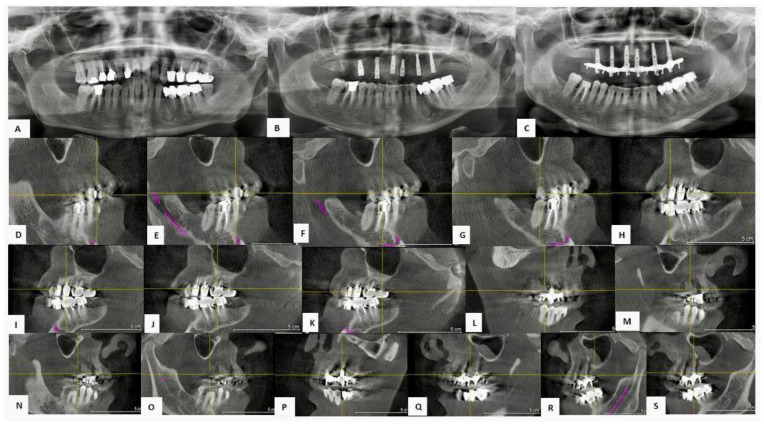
(A) Pre-operative OPT. (B) OPT check after sinus augmentation and after placement of six endosseous implants. (C) OPT check after 84 months. (D–K) Pre-operative CBCT sections of areas 1.4, 1.5, 1.6, 1.7, 2.4, 2.5, 2.6 and 2.7. (L–S) CBCT sections after 84 months of areas 1.4, 1.5, 1.6, 1.7, 2.4, 2.5, 2.6 and 2.7.
